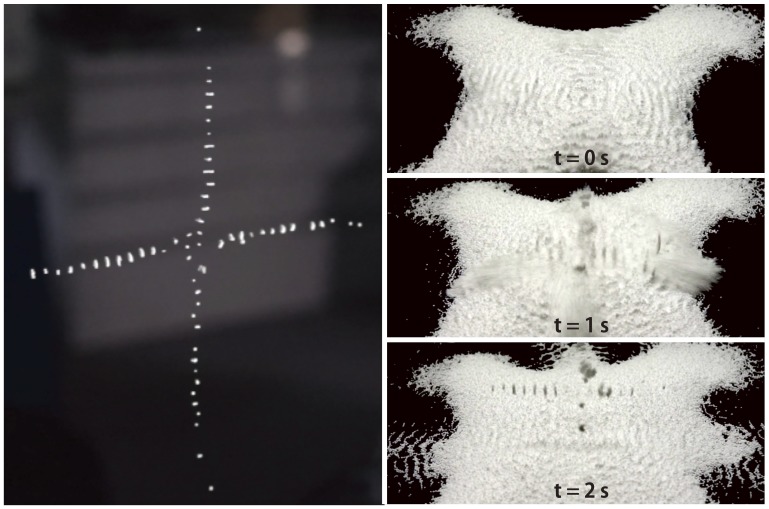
Manipulation of particles. (Left) Levitation and manipulation of particles with the vertical setup shown in Figure 3. (Right) Scooping up and holding particles with the horizontal setup in which all the ultrasonic beams are horizontally radiated.
